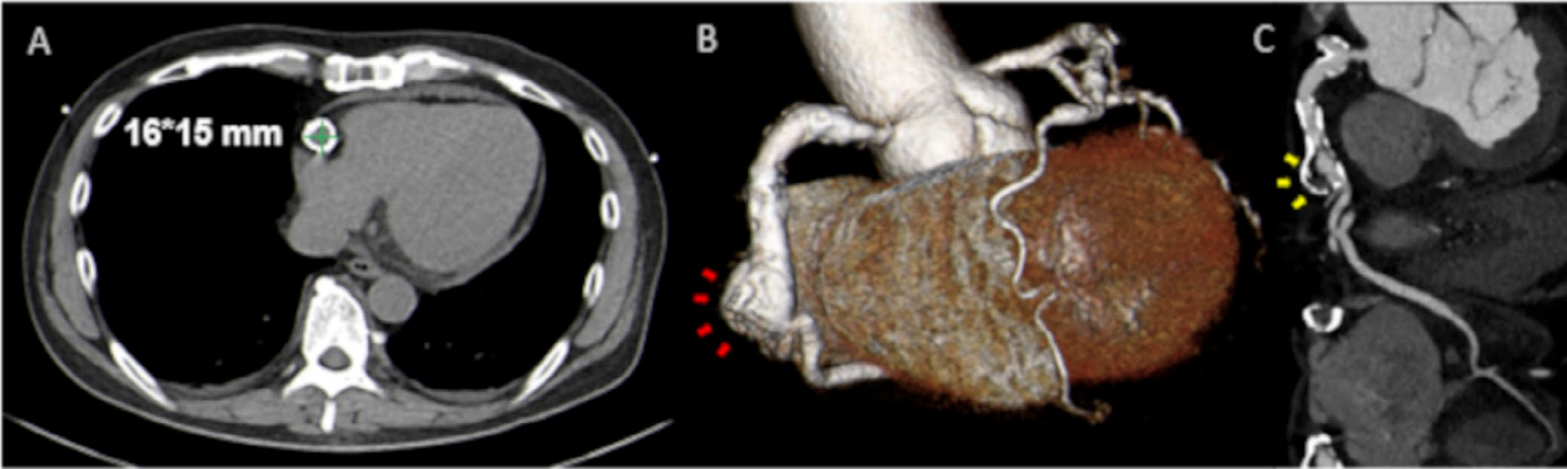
Cardiac computed tomography (CT) after percutaneous coronary intervention. (A) The 16 × 15 mm right coronary aneurysm revealed the entire circumference of calcification (green lines). (B) Cardiac CT demonstrated a saccular aneurysm (red arrows). (C) A saccular aneurysm demonstrated plaque and severe calcification (yellow arrows).
